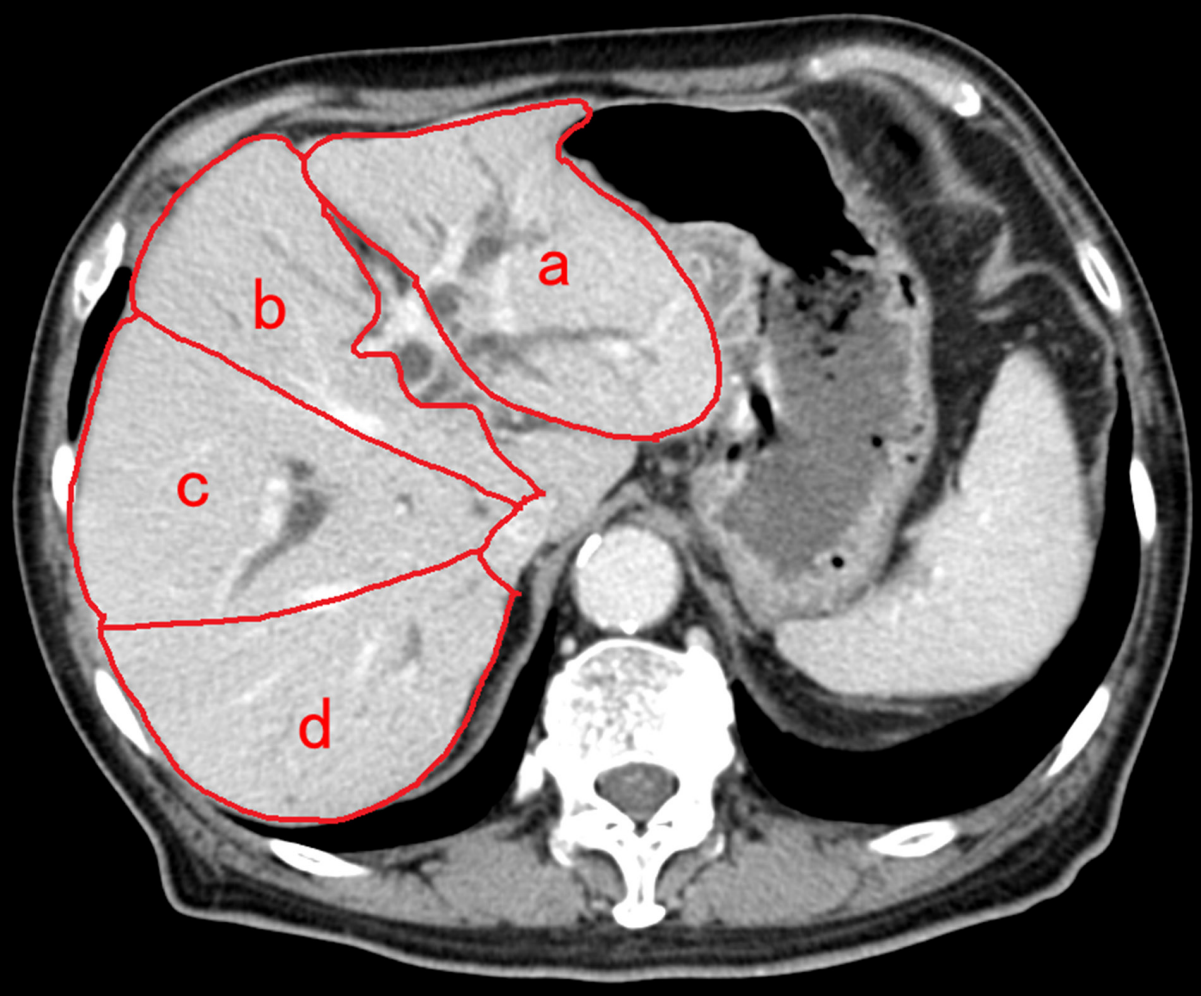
Method of identification of each liver section and computed tomography volumetry Four sectors of liver were defined based on the distribution of portal vein branches, excluding the caudate lobe. The areas of the left lateral sector (a), left medial sector (b), right anterior sector (c), and right posterior sector (d) were measured in computed tomography (CT) images. Additionally, the area of each sector was measured by tracing CT images, and then summing up the area of the CT slices comprising that sector.
